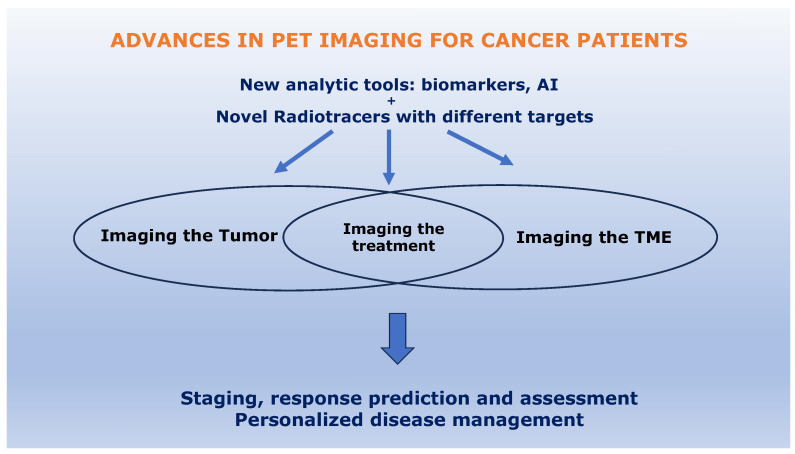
Strategies to improve PET/CT imaging of breast cancer patients.
